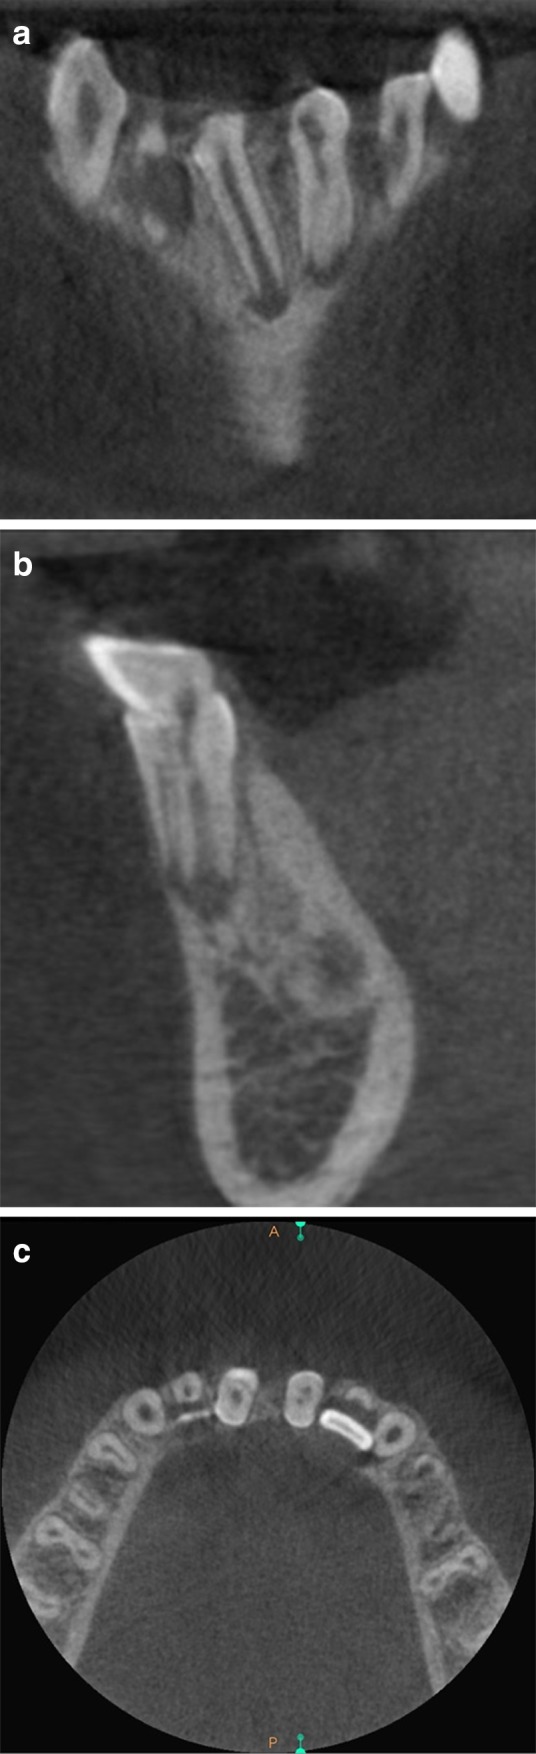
CBCT images of dens in dente anomaly of the left lower central incisor. a Tangential view. b Cross-sectional image. c Axial plane
